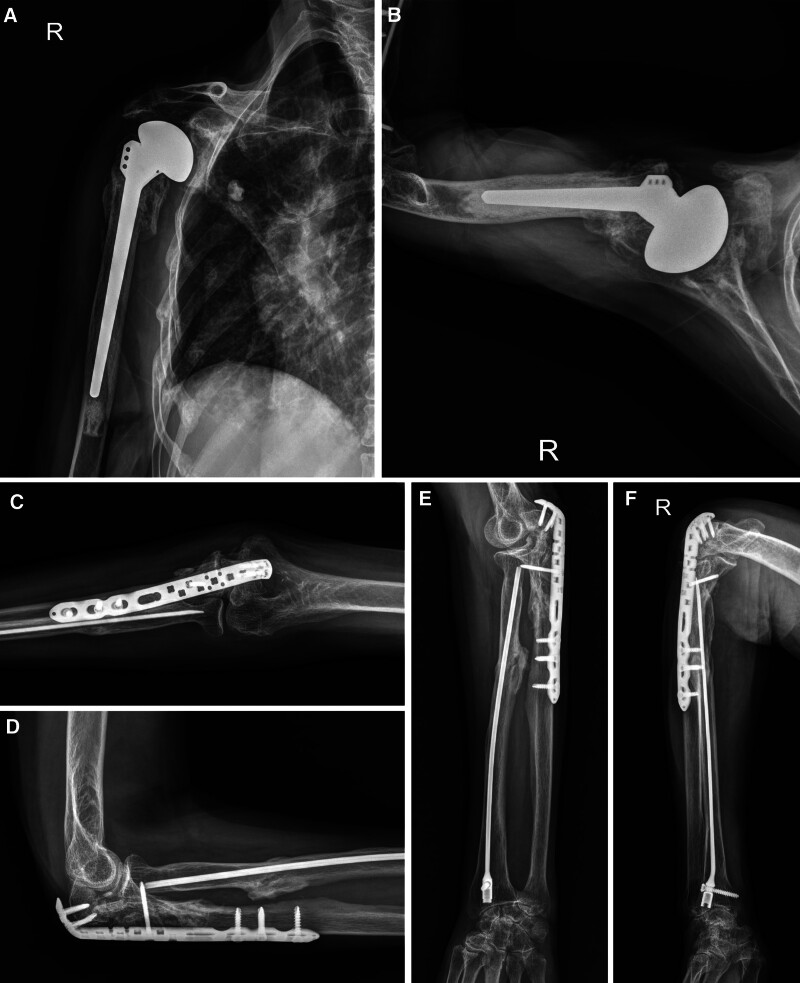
Patient’s post-operative plain radiographs at six months. (A, B) At 6 months, bipolar hemiarthroplasty was well maintained. (C–F) Open or closed reduction and internal fixation for the proximal ulna and radius fractures were also well maintained. Solid bone union was achieved and there were no complications at six months.
